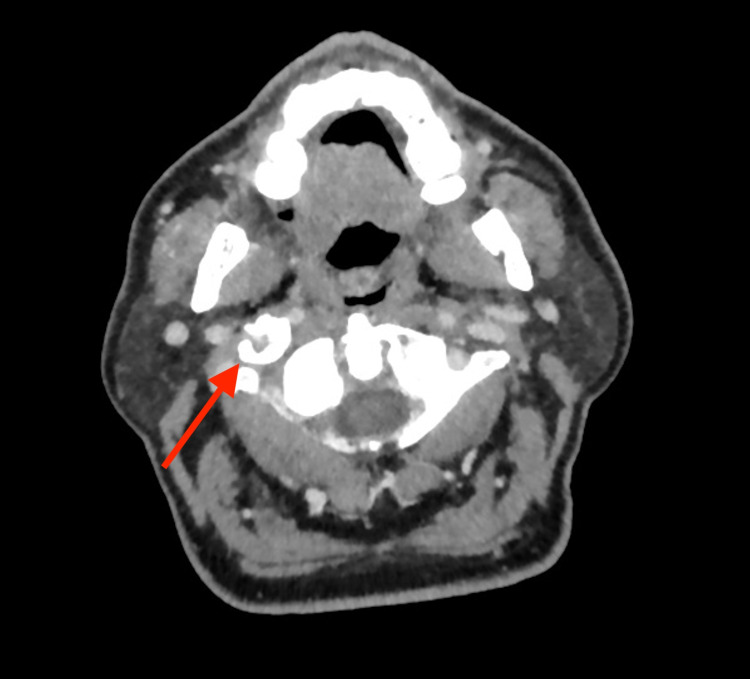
CT of the neck with contrast (axial plane) revealing a long, thick right styloid process (red arrow) consistent with a clinical diagnosis of Eagle's syndrome.
